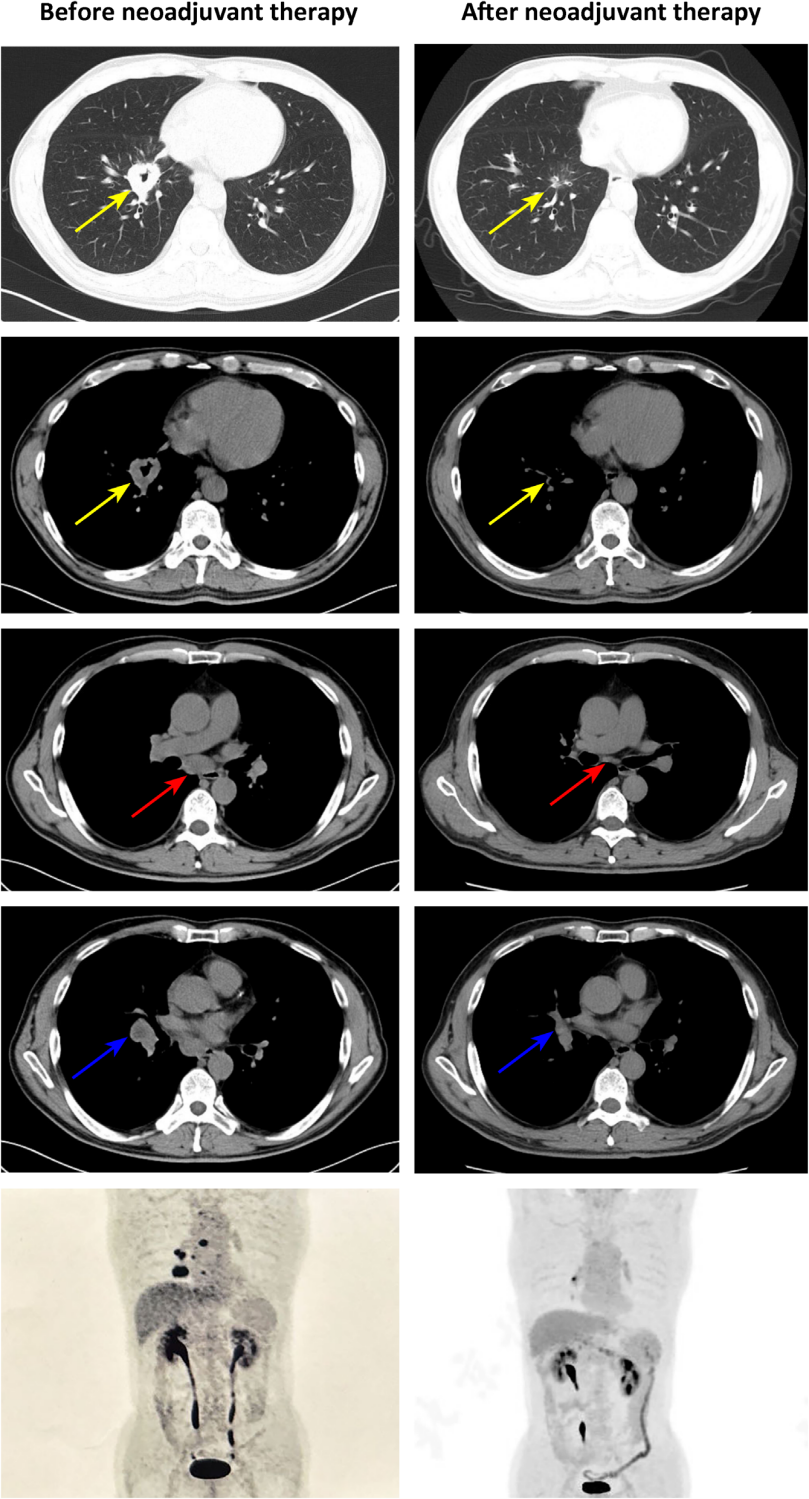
Imaging examination before and after neoadjuvant treatment. Chest computed tomography (CT) scan and positron emission tomography/CT (PET/CT) images of the right lung adenocarcinoma (yellow arrows), N7 (red arrows), and right hilar lymph (blue arrows) nodes before and after neoadjuvant treatment.
